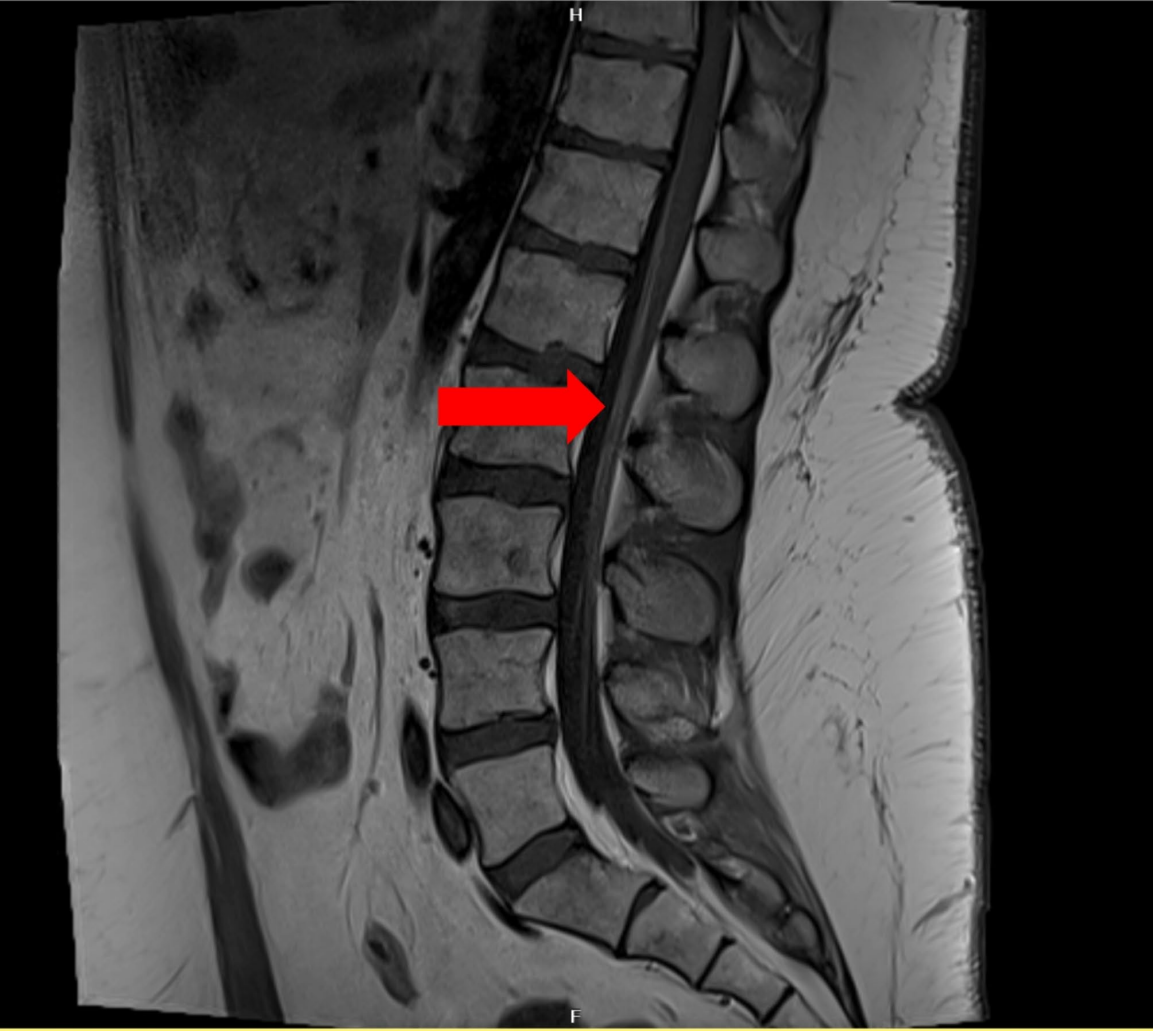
T1w-MRI with gadolinium of the lumbar spine steroid-responsive radiculopathy in Evans Syndrome (Arrow: gadolinium enhancement)
